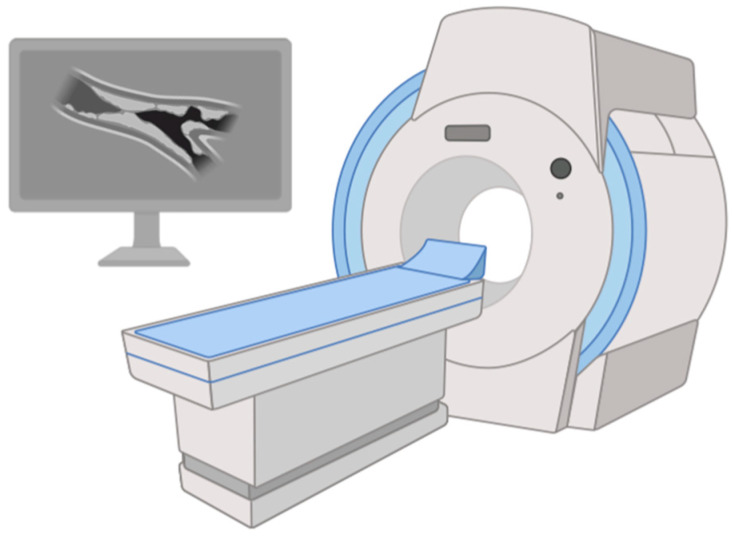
An example of MRI equipment for setup of atherosclerosis plaque imaging.
